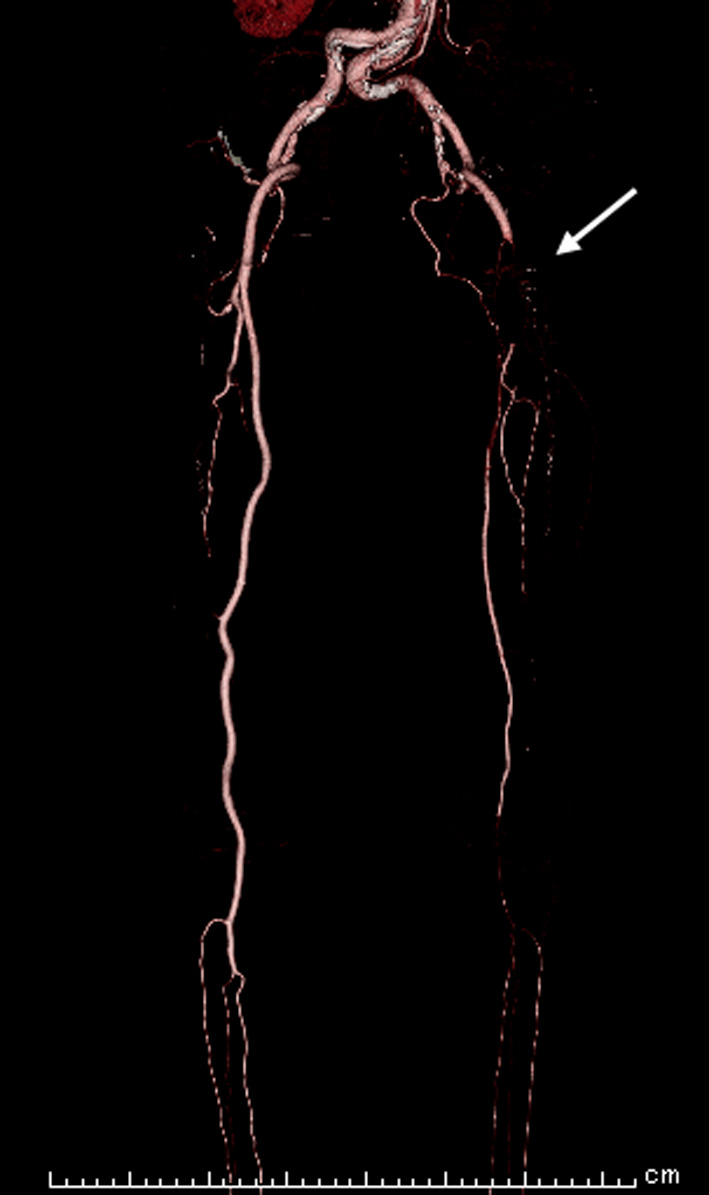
Preoperative computed tomography. Preoperative computed tomography shows thrombi in the left common femoral, superficial and deep femoral, and popliteal arteries (arrow)
